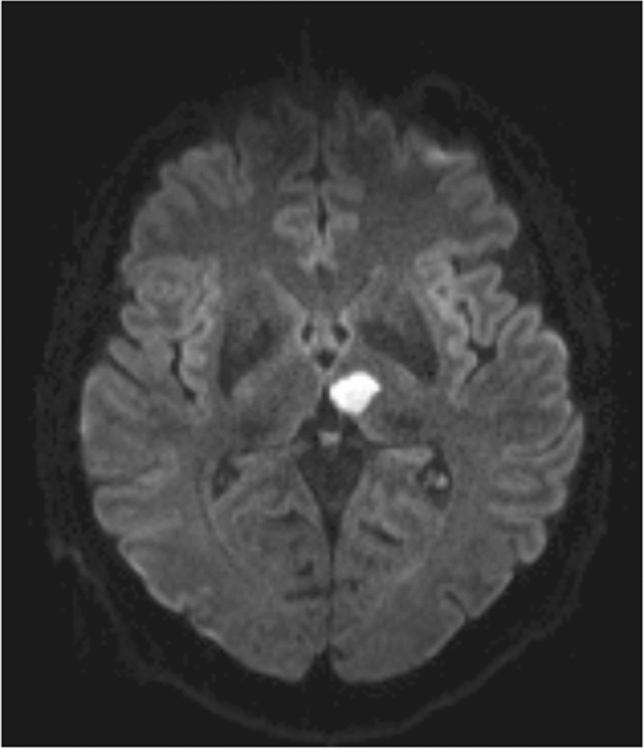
Example of a unilateral, paramedian ischemic lesion in the left thalamus (DWI imaging)
